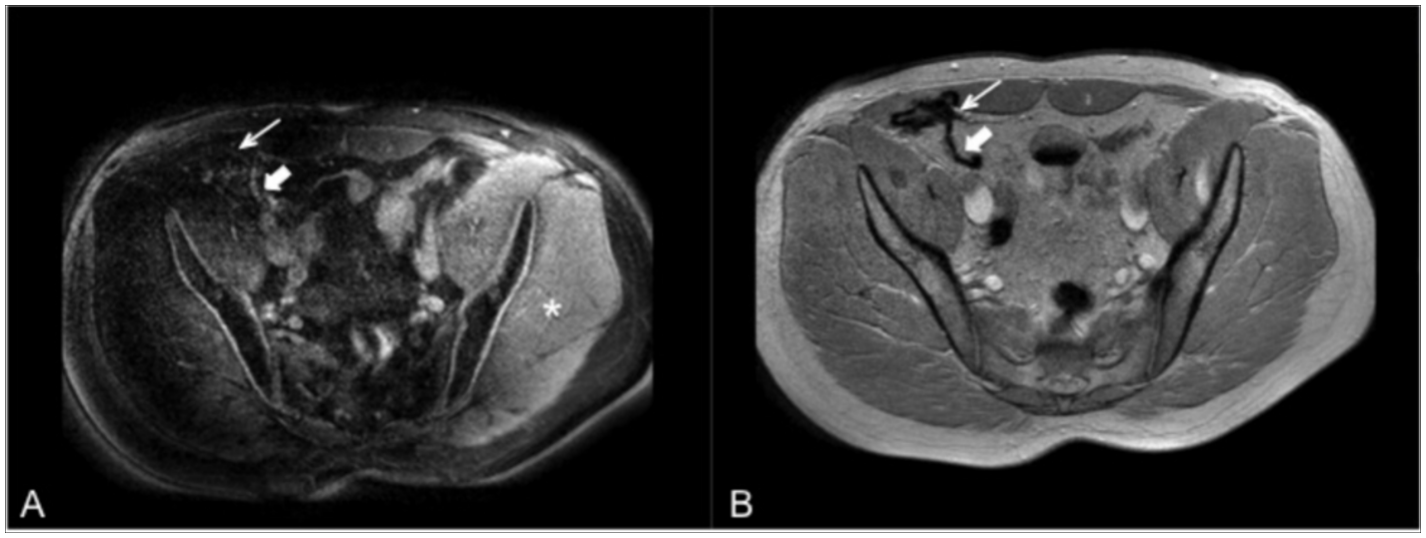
Transversely orientated MR images with a heavily folded mesh configuration (pronounced in the ventral mesh parts). On PCSI (A) the mesh can be discerned when configurated even (thick arrow), but fails to exhibit positive contrast when heavily folded (slim arrow). On GRE images (B), the mesh can be depicted as signal voids irrespective of its configuration (thick/slim arrows). On PCSI (A), B1-inhomogeneties result in inhomogenous background suppression (asterisk, *). On GRE (B), this effect is not present.
